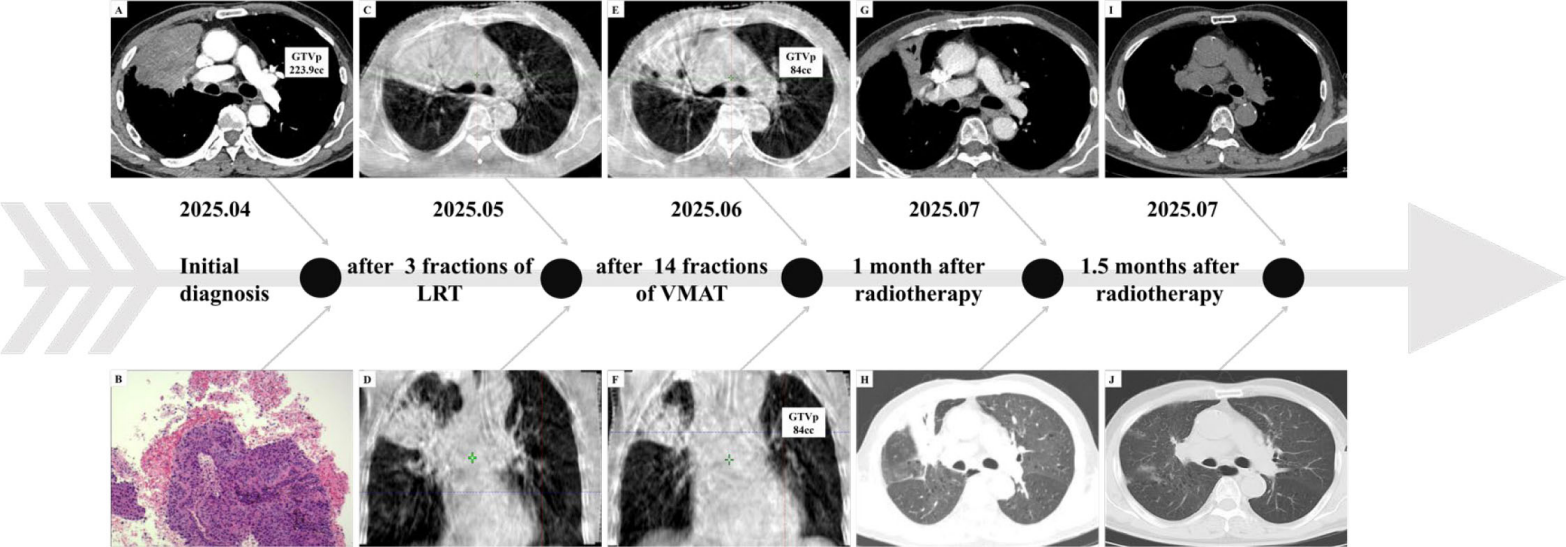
Timeline of treatments. (A) The initial chest CT detected a pulmonary tumor. (B) Pathology of the right upper-lobe tumor. (C, D) On-treatment CBCT after 3 fractions of LRT. (E, F) On-treatment CBCT after 17 fractions (3 LRT + 14 VMAT). (G, H) Chest CT 1 month after completion of 31 fractions of radiotherapy. (I, J) Chest CT 1.5 months after radiotherapy at follow-up.
